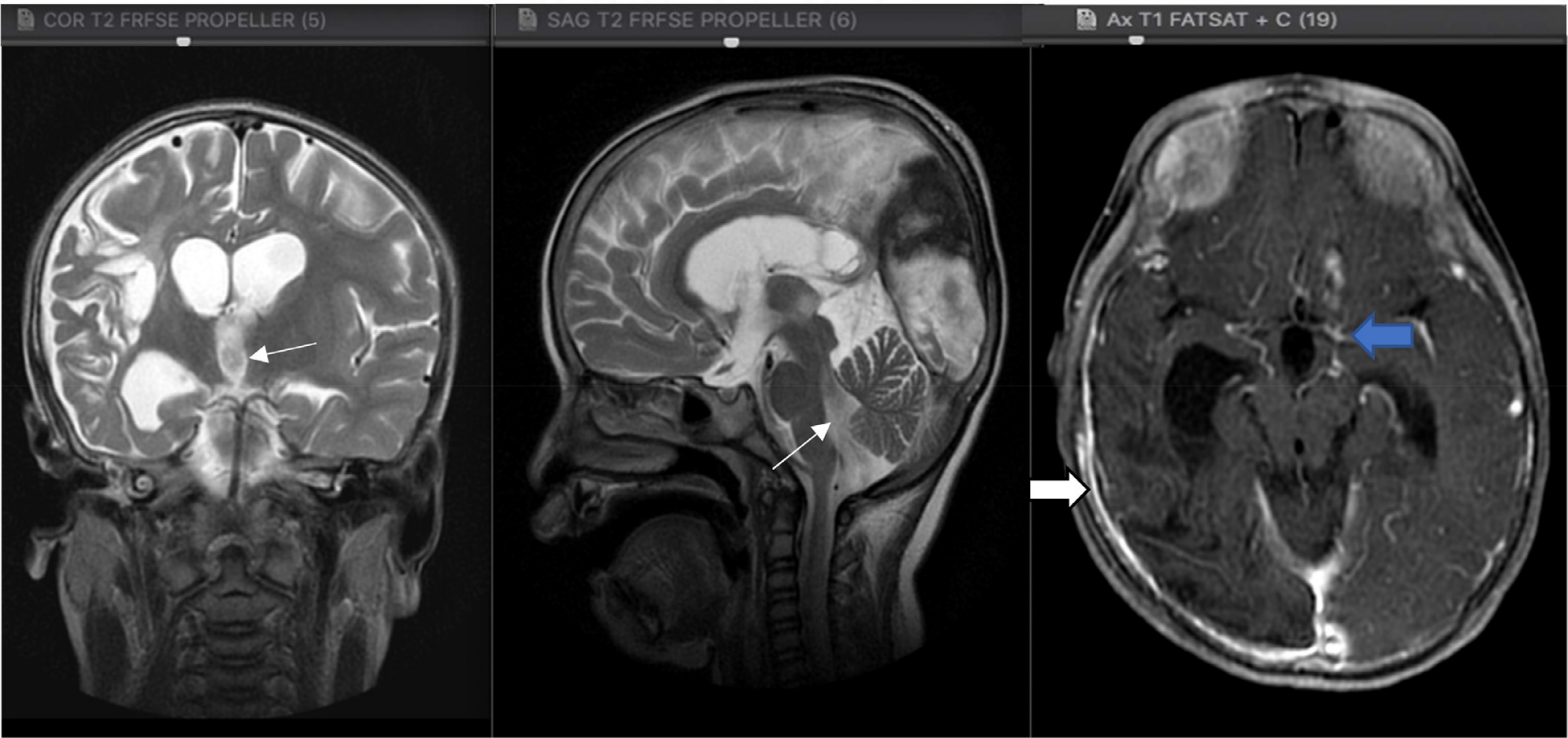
The coronal and sagittal section of the T2 MRI, dilation of the III and IV ventricles (white thin arrow) with pachymeningeal enhancement in the right temporo-parieto-occipital region (white arrow), and from axial section of the T1 fatsat MRI, the enhancement in the left cisterna basalis on contrast administration (blue arrow).
